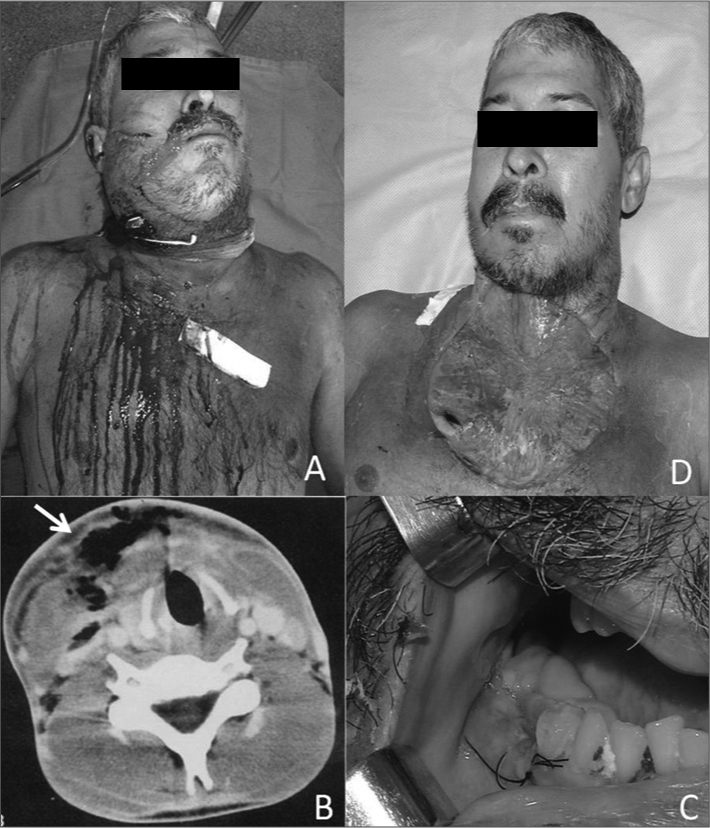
A) Extensive erythematous area associated with bilateral submandibular, submentonian, and sublingual edema extending to the neck and thorax. B) Subcutaneous emphysema of the neck - computed tomography. C) Removal of many teeth roots in the mandible (primary infection sites). D) The surgical wound on the sixth post-operative day. Note the extensive open area and residual cavity with pus.
